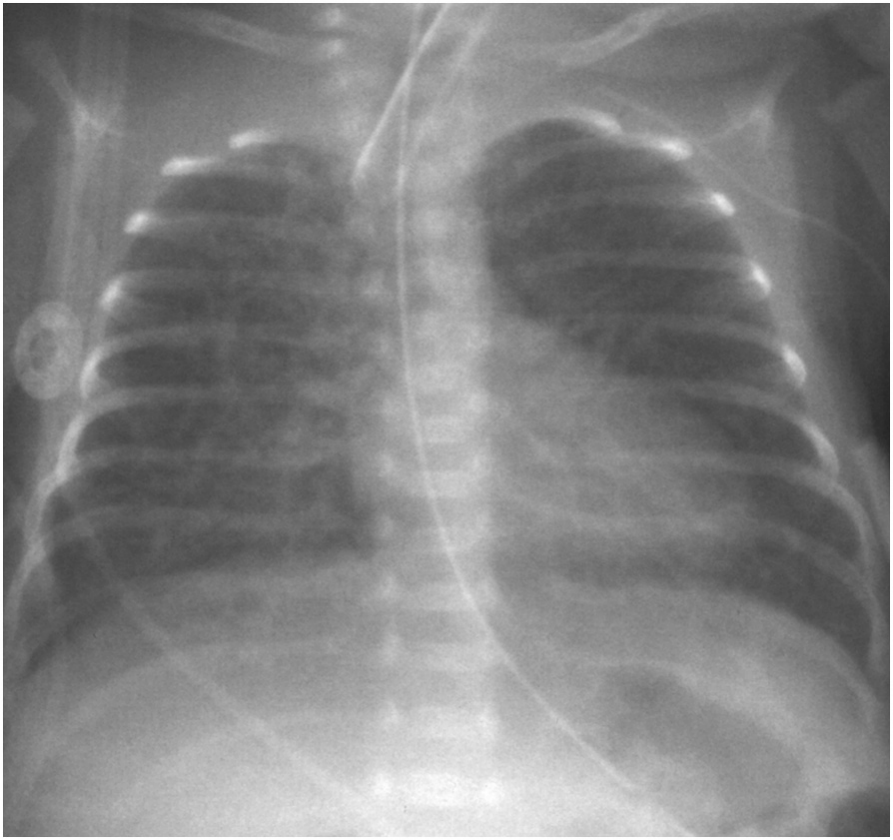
A chest X-ray obtained on postnatal day 37. Almost complete regression of pulmonary interstitial emphysema can be seen.
